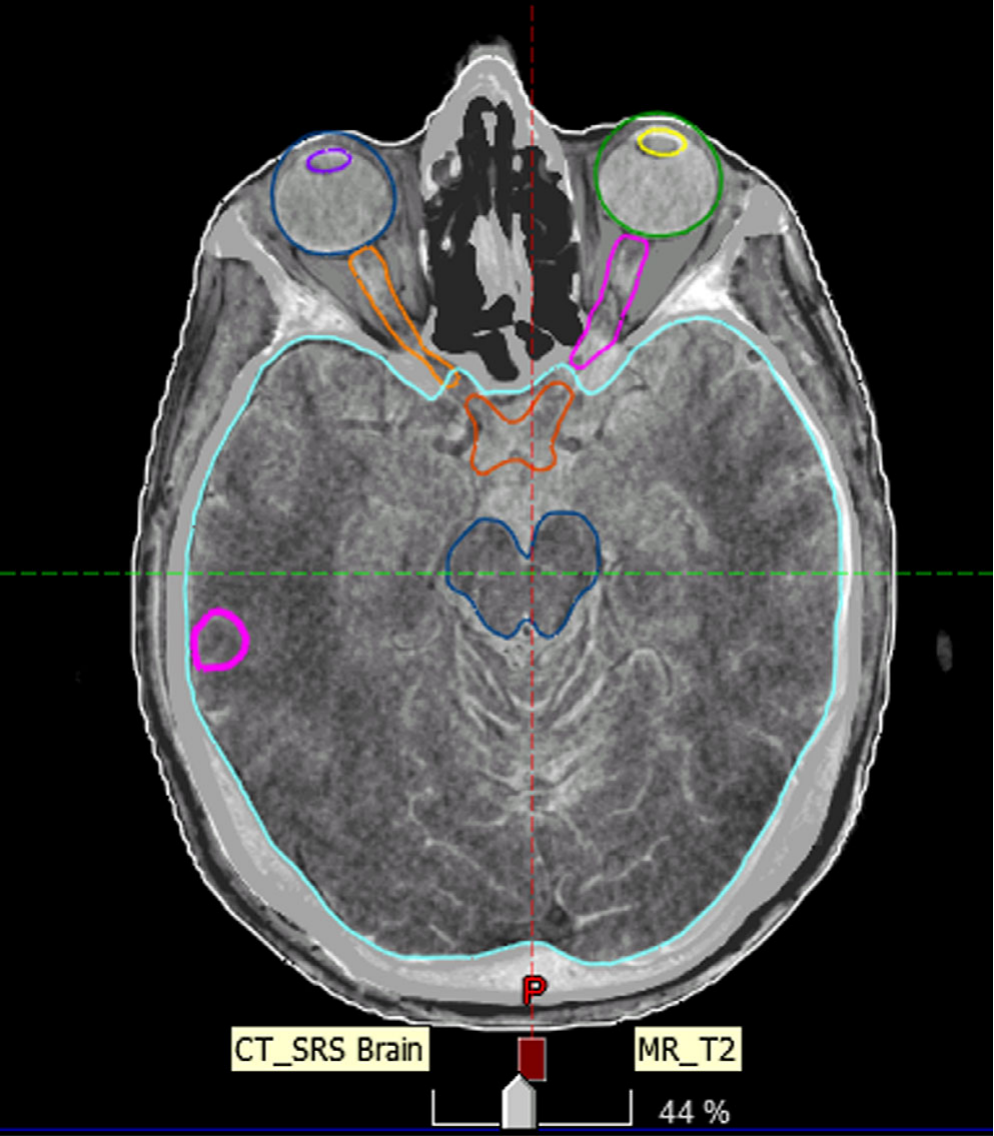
Registered CT and MR images were used to contour the normal structures and targets.
